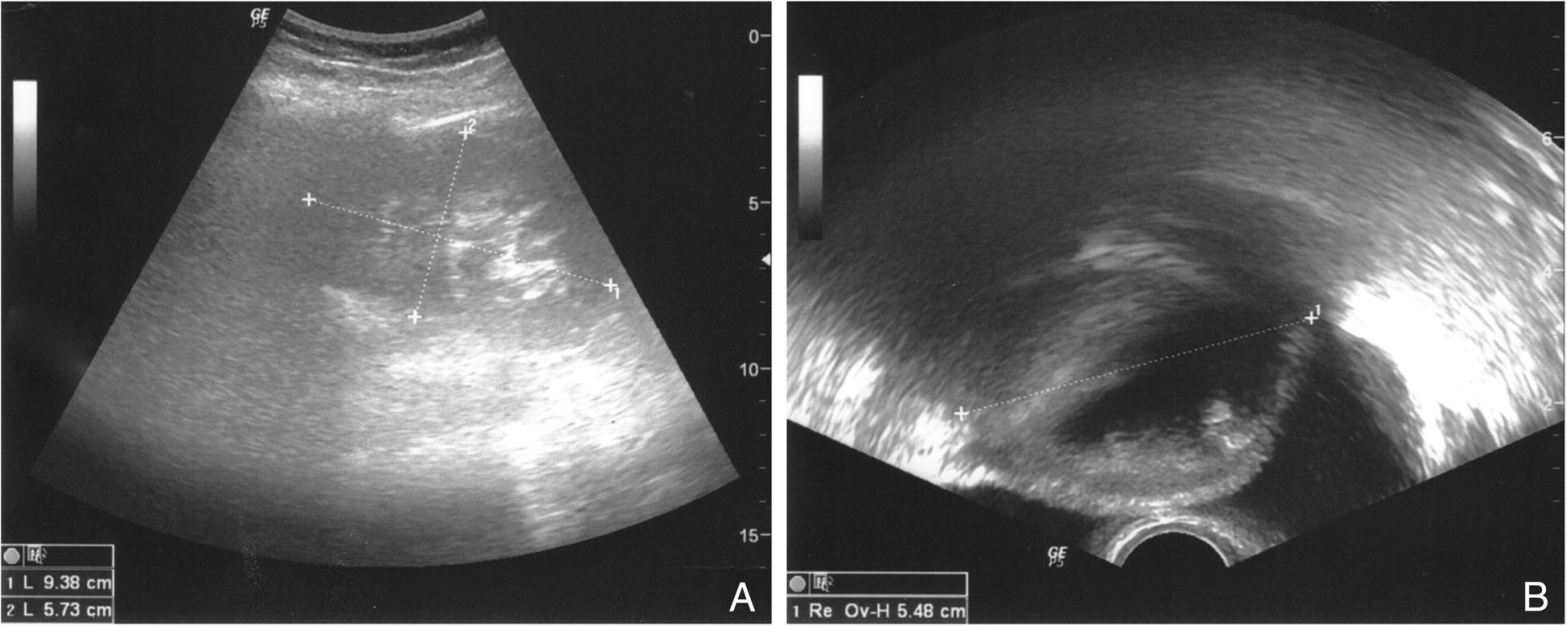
a Transabdominal ultrasound. b Transvaginal ultrasound shows the suspected tumor in the lower abdomen, measuring about 5.5 × 5.7 × 9.4 cm. The cyst has a solid as well as fluid content and an anechoic area behind the cyst. The bladder is not involved
